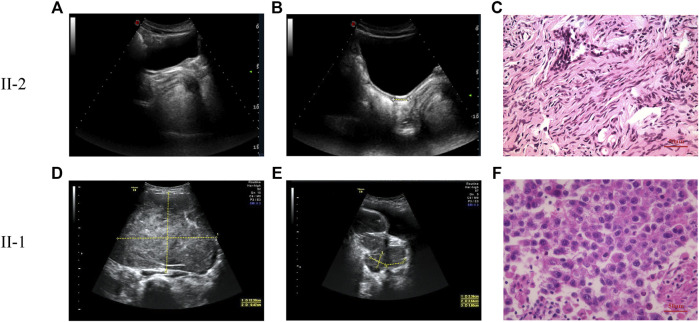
The pelvic ultrasound and pathological images of patients. (A,B) The pelvic ultrasound of proband (II-2). Yellow dashed lines indicate the length of ultrasonography. (A) No identifiable testicular or ovarian tissue was observed in the pelvic ultrasound. (B) Underdeveloped uterus (1.5 × 0.5 × 1.0 cm) of the proband. (C) Pathological section analysis of proband (HE, ×40). Pathological evaluation reveals that the bilateral streak gonads are ovarian interstitial-like cells and germinal epithelium, without any malignant transformation. (D,E) The pelvic ultrasound of patient 2 (II-1). Yellow dashed lines indicate the length of ultrasonography. (D) Left ovarian mass (12.2 × 9.5 × 7.0 cm). (E) Right ovarian mass (2.3 × 2.6 × 1.8 cm). (F) Pathological section analysis of patient II-1 (HE, ×40). The pathological evaluation identified the bilateral ovarian tumors as dysgerminoma.
